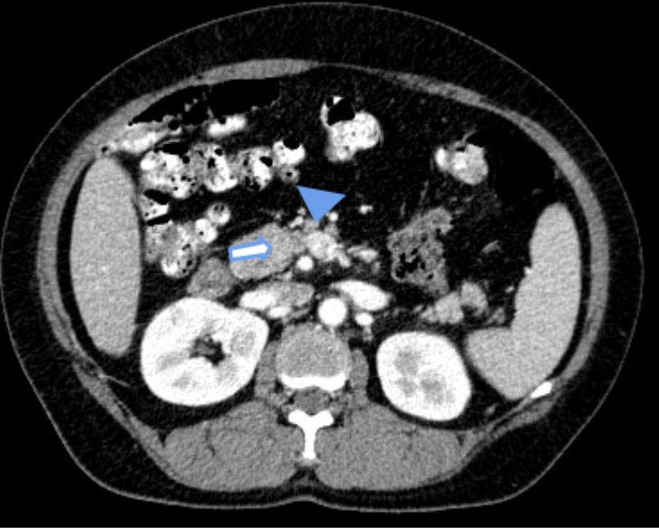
Contrast enhanced CT scan of the abdomen shows SMA (block arrow) lying on the right side of SMV (arrowhead) revealing an inverse relationship between them.
